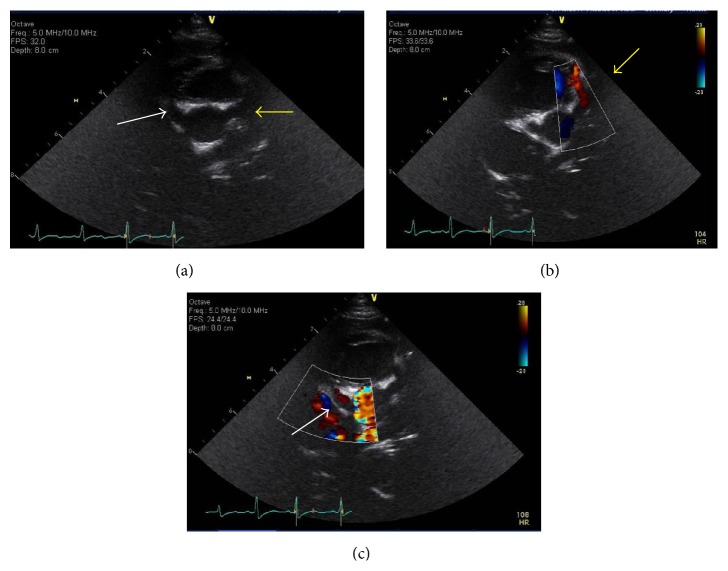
Presurgical transthoracic echocardiographic images of the right and left coronary arteries. (a) 2D transthoracic echocardiographic coronary images prior to cardiac surgery demonstrate dilated proximal right coronary artery (white arrow) and left main coronary artery (yellow arrow). (b) 2D and color Doppler transthoracic image of the dilated left main and left anterior descending coronary arteries (yellow arrow). (c) 2D and color Doppler transthoracic image of the dilated right coronary artery (white arrow).
